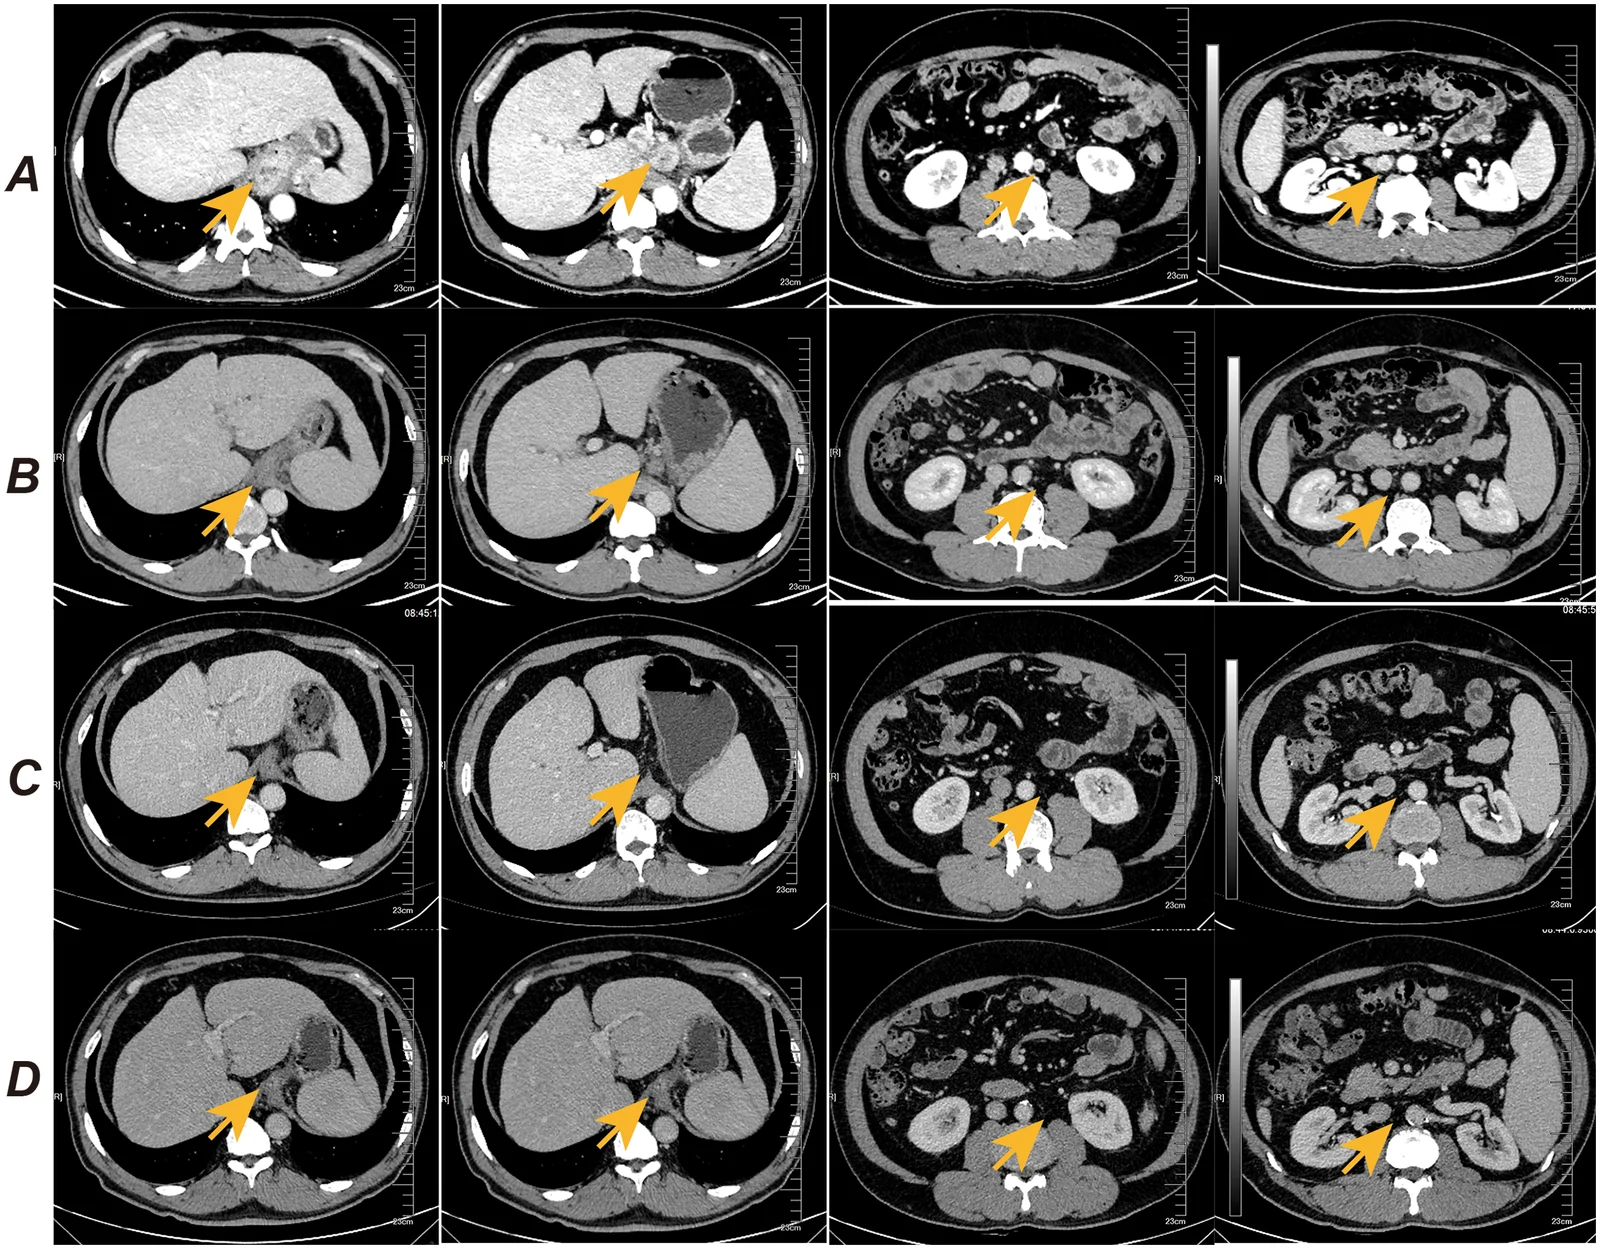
Radiographic evolution of tumor burden during the treatment course. (A) Baseline CT scans showing the primary esophagogastric junction tumor, perigastric and retroperitoneal lymph nodes. (B) After two cycles of XELOX chemotherapy, showing a partial response with significant tumor reduction. (C) At the time of brain metastasis diagnosis (May 2022), showing stable extracranial disease without systemic progression. (D) Most recent follow-up (October 2025), demonstrating a sustained complete response with complete resolution of all lesions.
